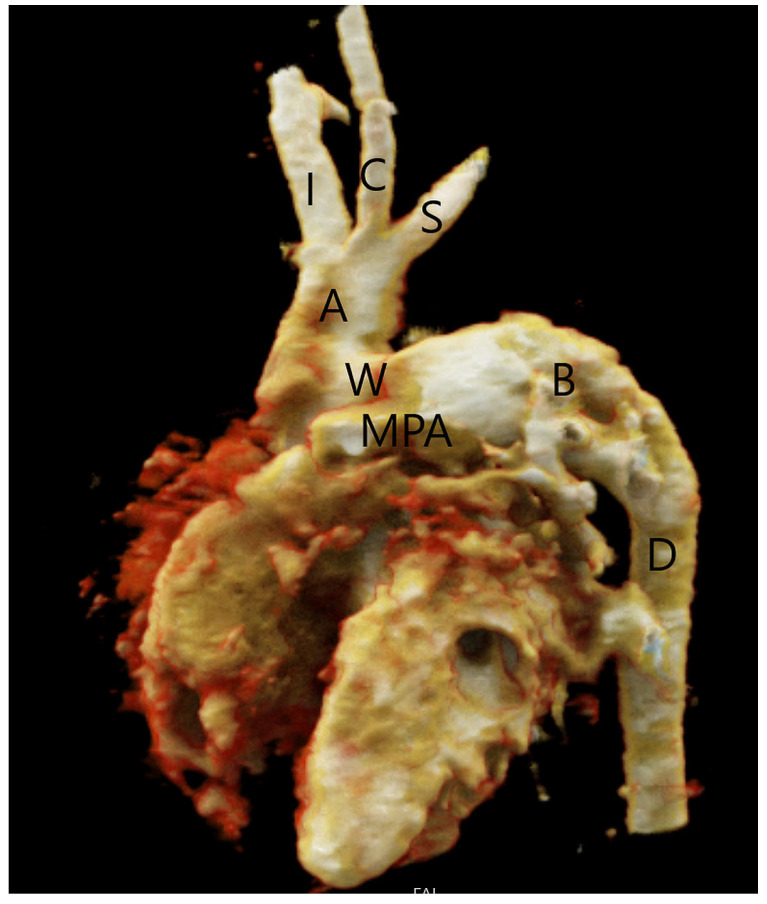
Type A interrupted aortic arch in a patient with aortopulmonary septal defect type 2 (W), volume rendering reconstruction of CT angiography. The aorta (A) ends distally to the origin of the left subclavian artery (S). The descending aorta (D) is supplied by the patent ductus arteriosus (B). Main pulmonary artery (MPA), innominate artery (I), and left common carotid artery (C).
